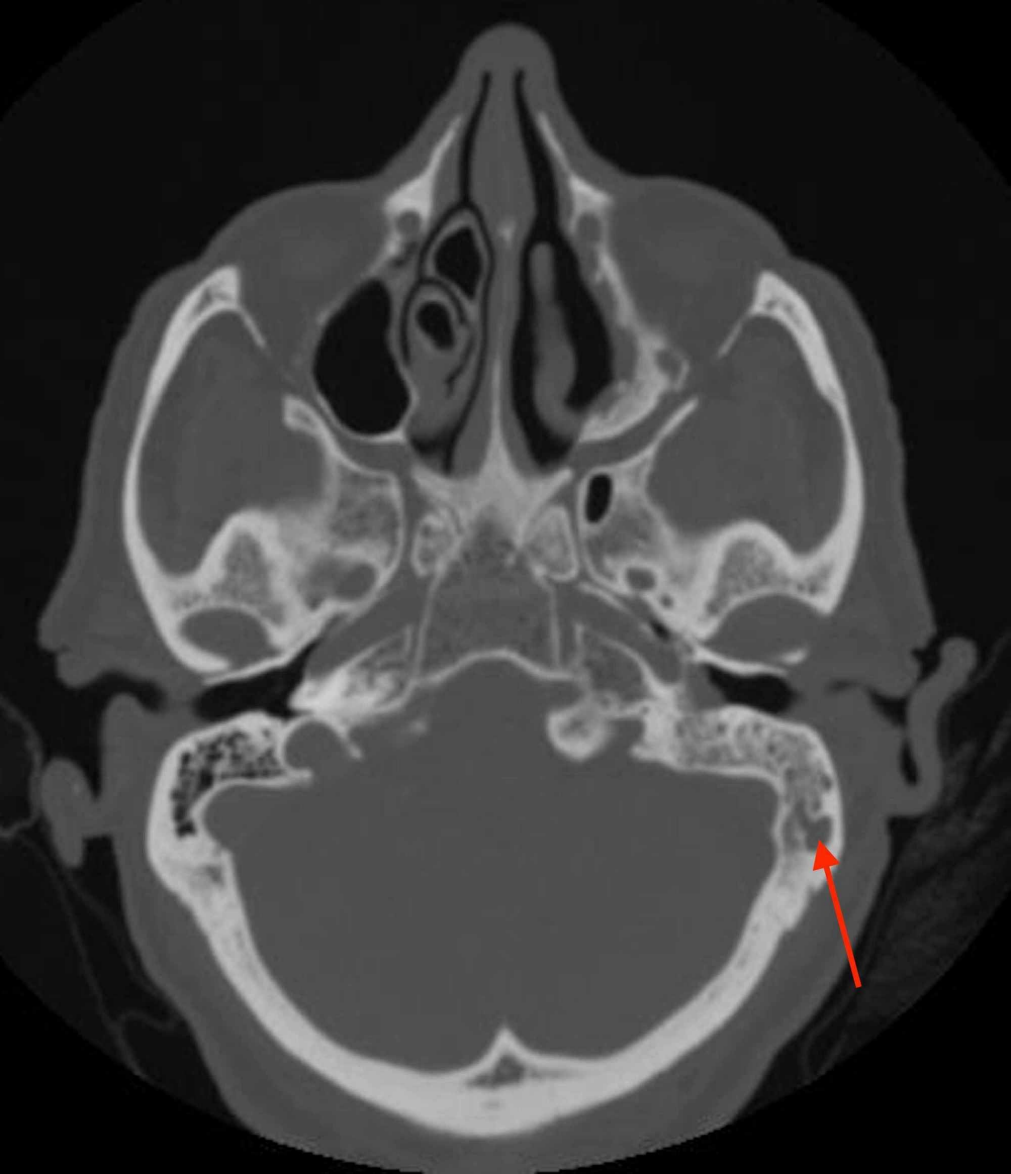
Axial temporal computed tomography. The red arrow is pointing at opacification in the left mastoid air cells.
